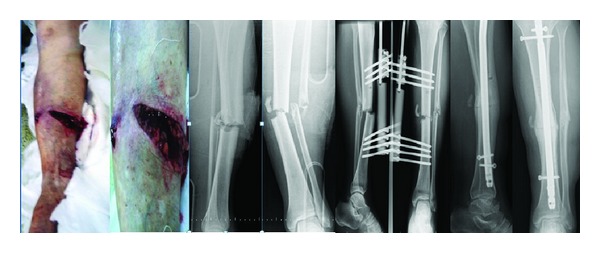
Case 11: AO/OTA: 42B2, GA: 3C, 43-year-old female, traffic accident, second stage: vessel repair, third stage: IMN, 15-month followup, X-ray: full union.
